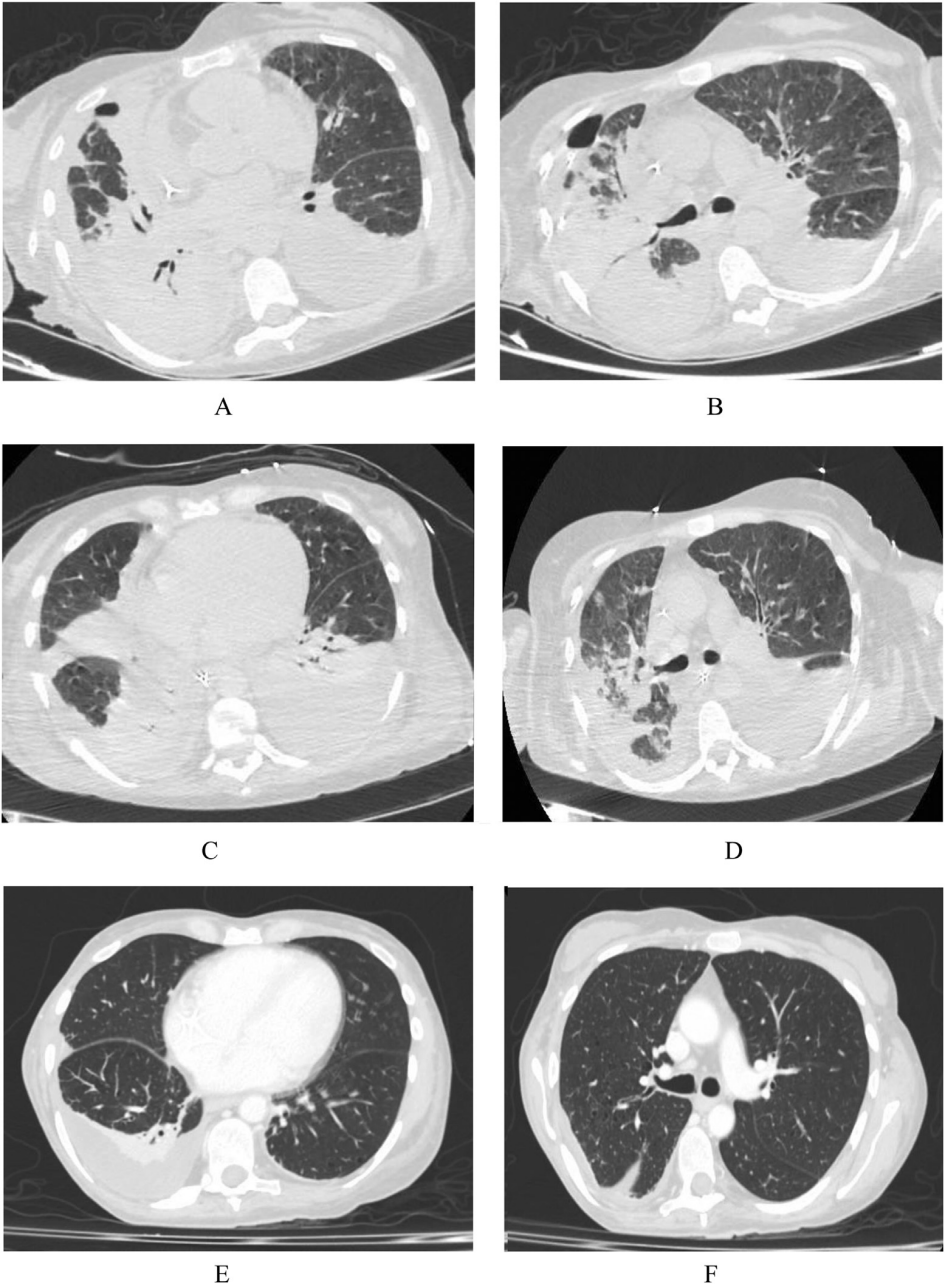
The patient's chest CT images. (A-B) (before treatment) show multiple bilateral areas of increased parenchymal density, partly "ground glass" and partly consolidative, with bilateral pleural effusion. (C-D) (under treatment). (E-F) (after treatment).
